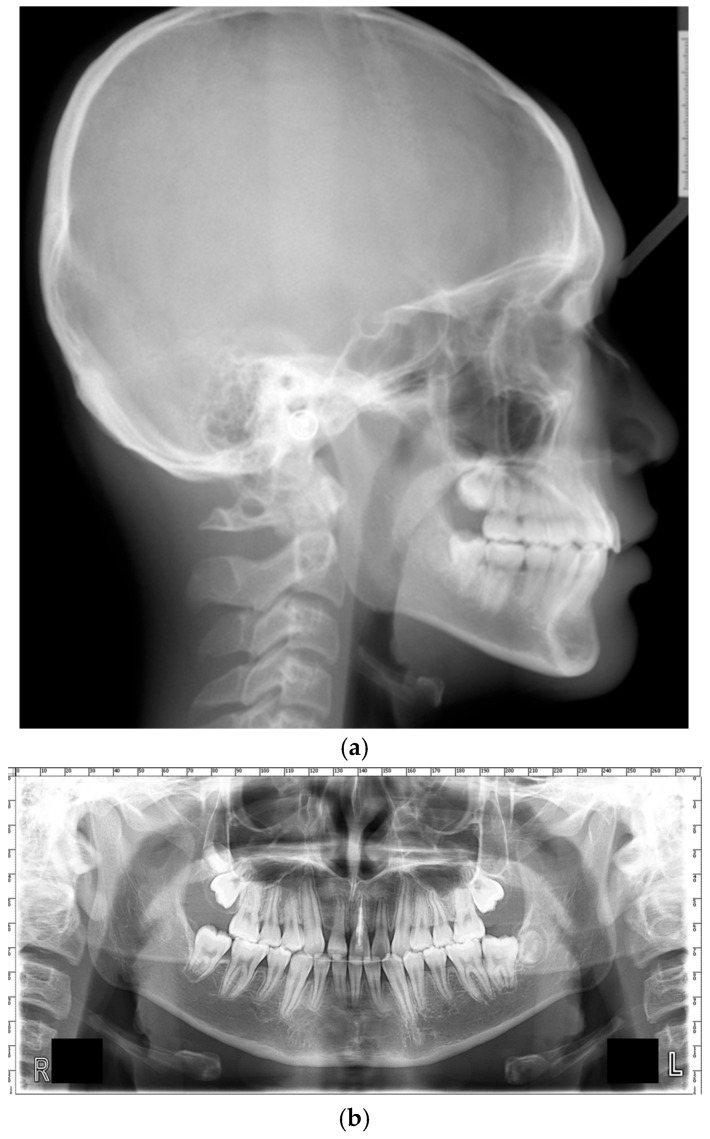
Cephalometric (a) and panoramic (b) X-rays at the end of the orthodontic treatment, July 2023.
